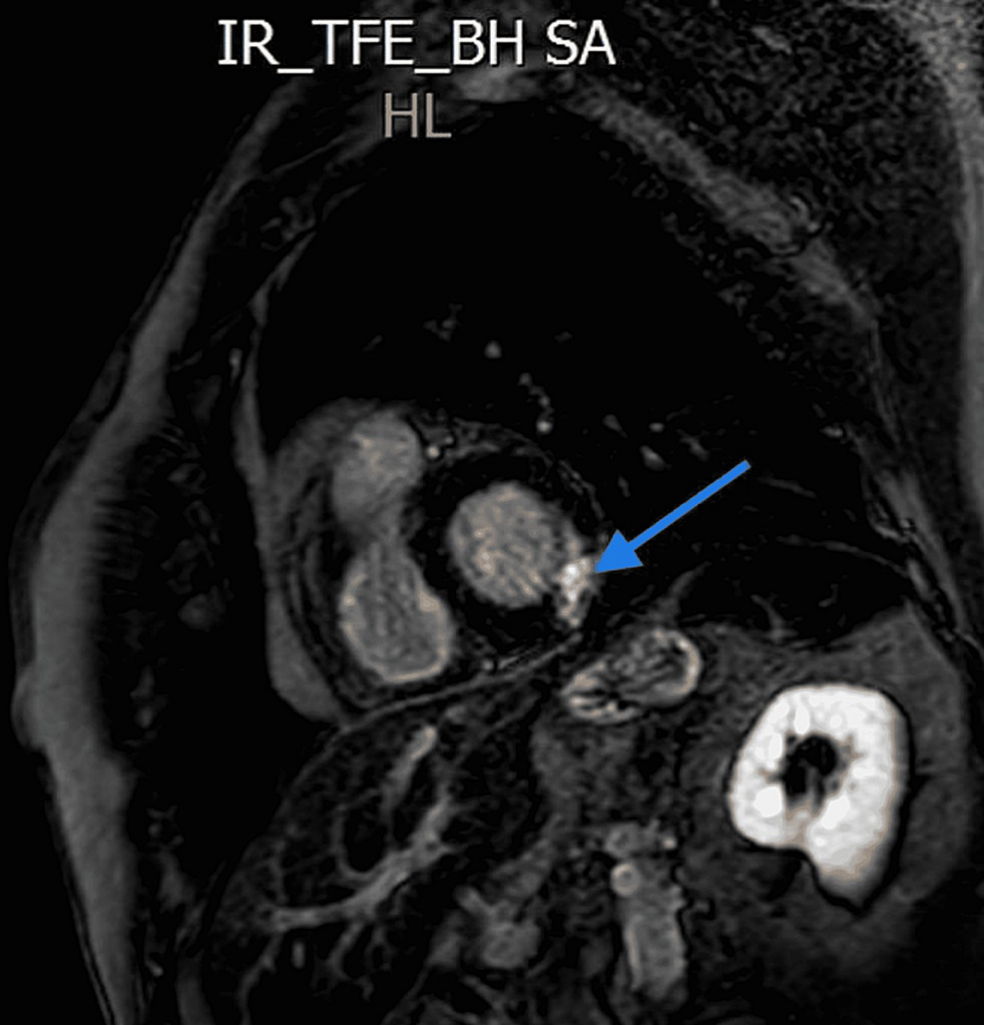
MRI T2 sequence showing mid myocardial increased signal in the inferolateral wall suggesting myocarditis (blue arrow).
